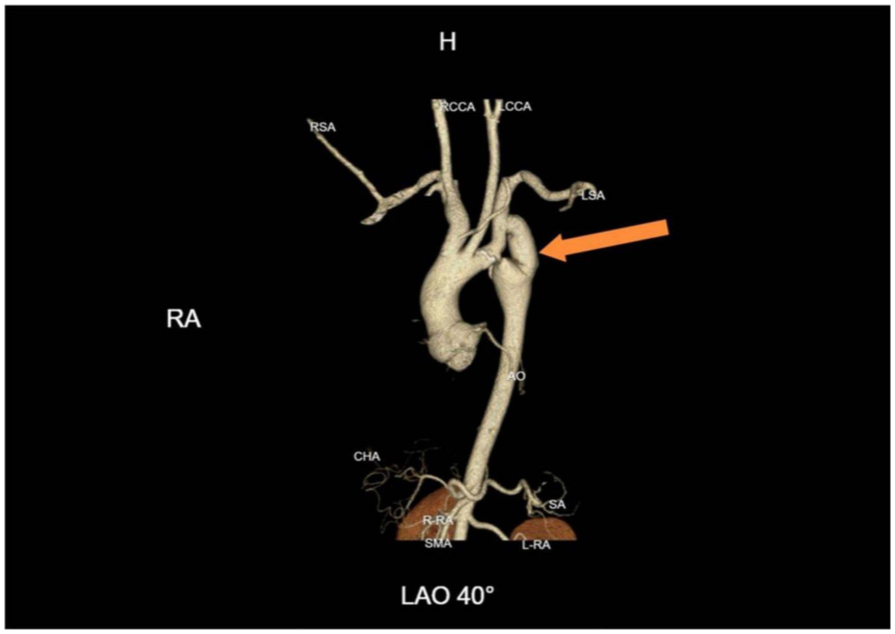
One-year follow-up CTA demonstrating graft patency. Continued patency of the bypass graft with no evidence of pseudoaneurysm, graft occlusion, or aneurysmal progression at the coarctation site.
